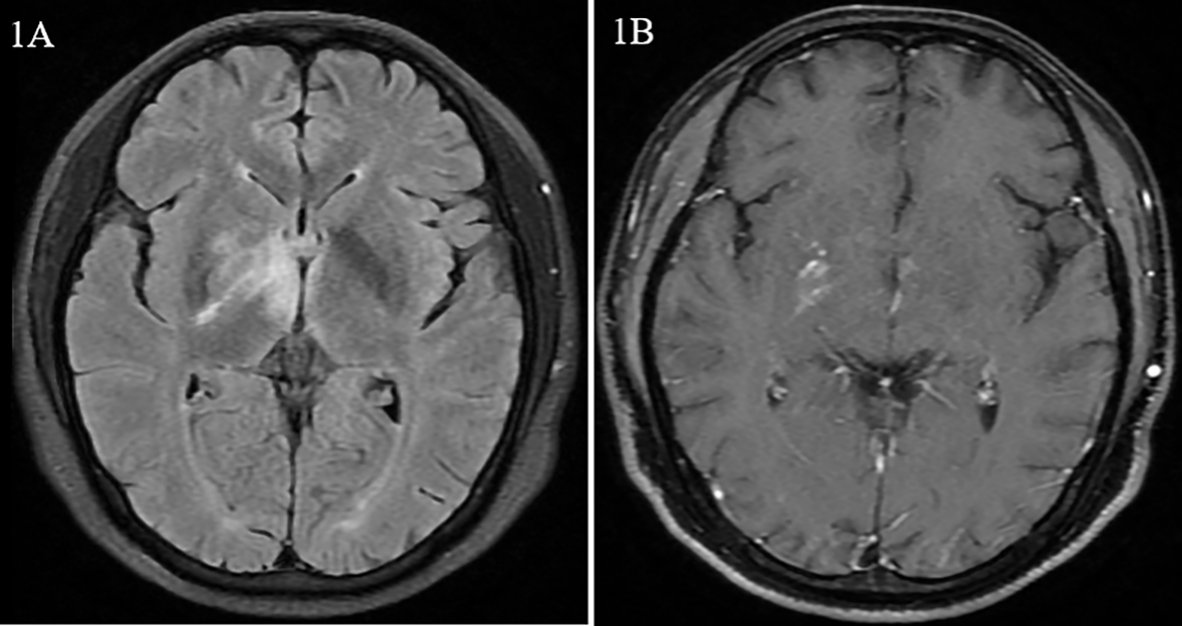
Magnetic resonance images from case 1. (A) Hypersignal of the right basal ganglia and thalamus on fluid-attenuated inversion recovery (FLAIR). (B) Nodular enhancement of the right cerebral peduncle on dynamic enhanced magnetic resonance imaging.
